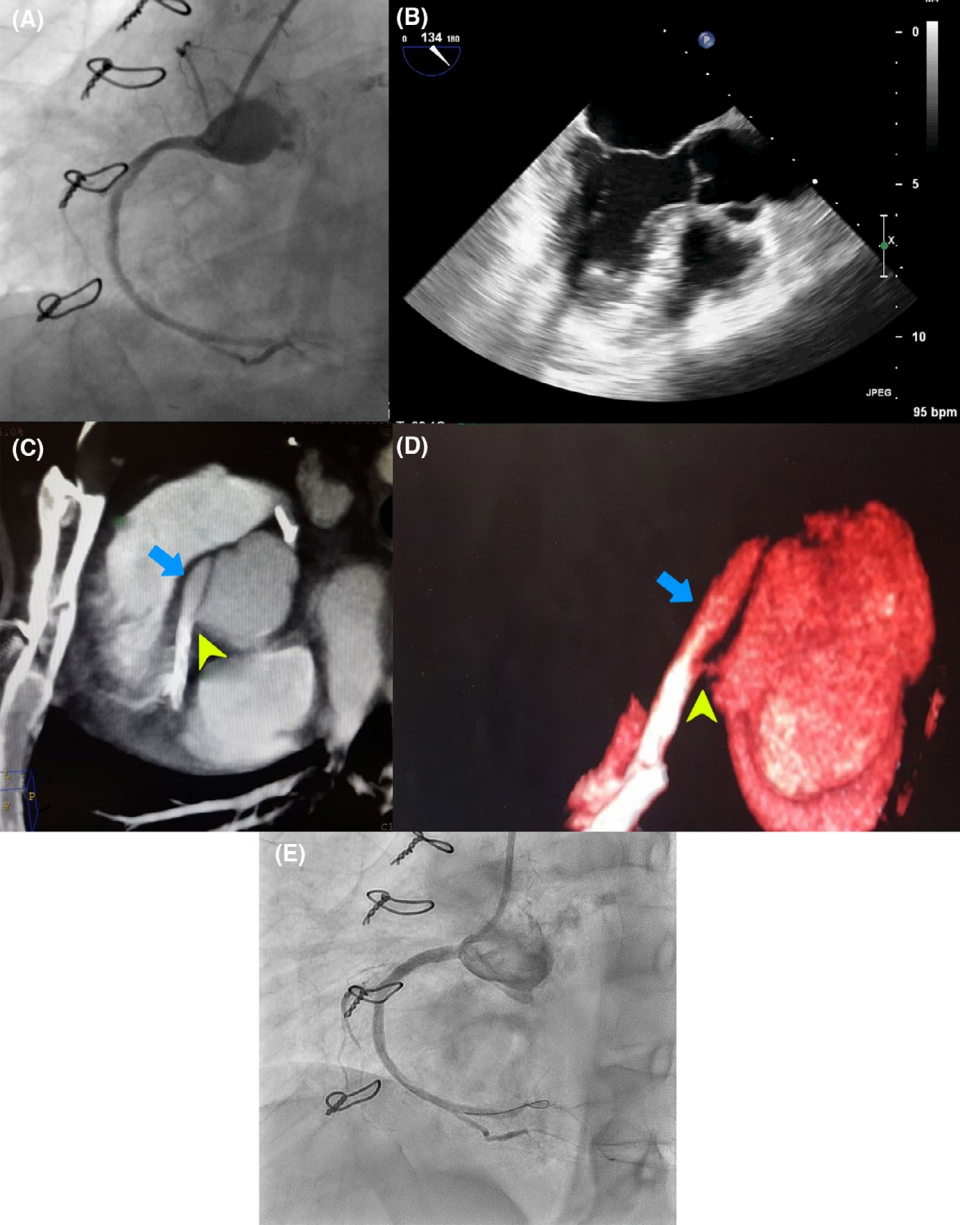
A, Right coronary injection showed a significant pseudoaneurysm at the proximal portion of RCA. B, Transesophageal echocardiography revealed thick intimal flap in the right sinus of Valsalva possessing flow within. C and D, Coronary CT angiography confirmed the presence of pseudoaneurysm at the proximal portion of RCA (blue arrows indicate pseudoaneurysm, and yellow arrowheads indicate the origin of pseudoaneurysm). E, Pseudoaneurysm was sealed off after the deployment of a covered stent at the ostium of RCA with acceptable final result
